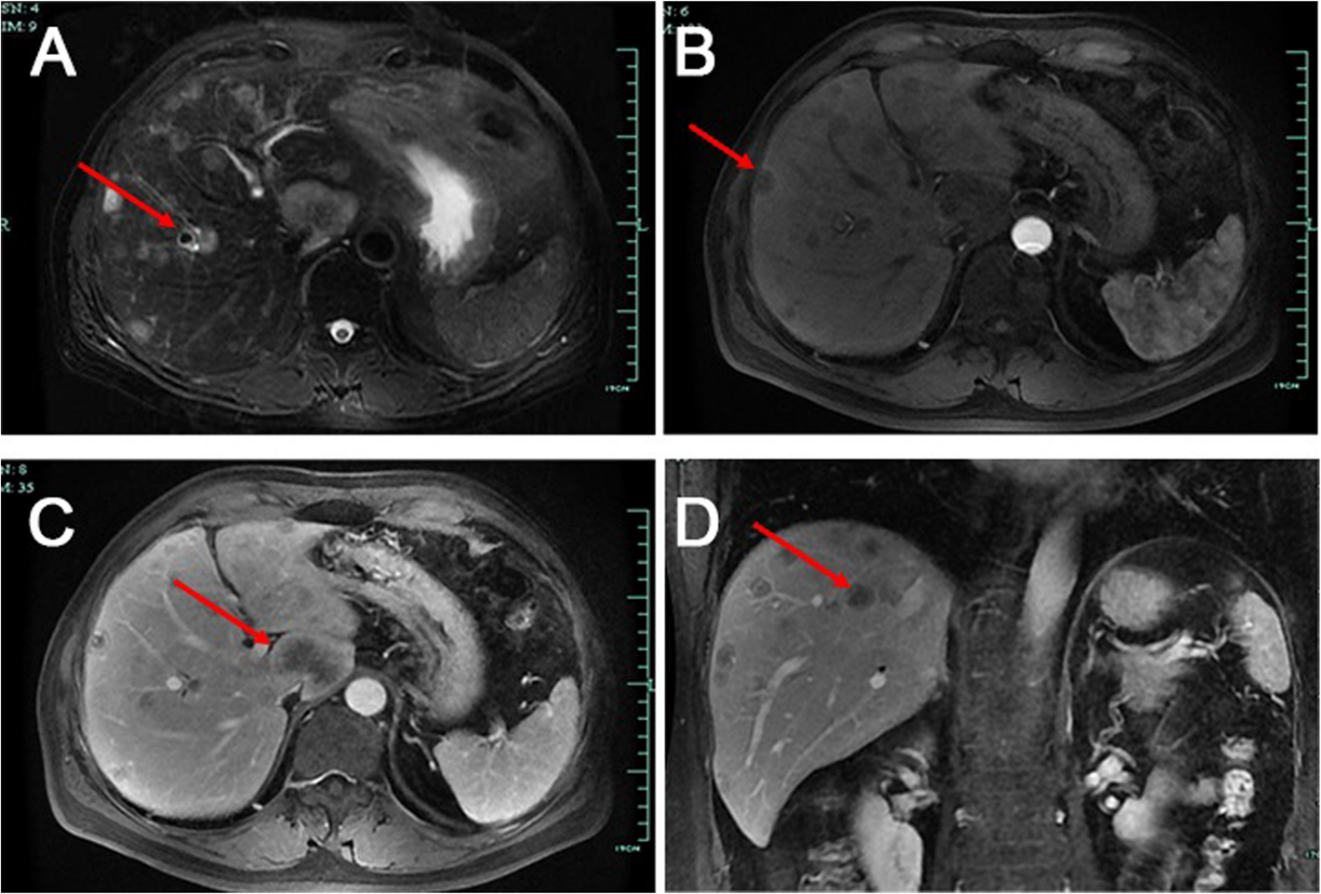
MRI scan images of a hepatic epithelioid hemangioendothelioma patient (arrows). a T2-weighted MRI showing multiple lesions with low signal. b Artery venous phase showing multiple lesions with hyperintensity. c Portal venous phase showing multiple lesions with a heterogeneous mild to moderate hyperintensity. d Coronal view image showing multiple lesions in the liver
